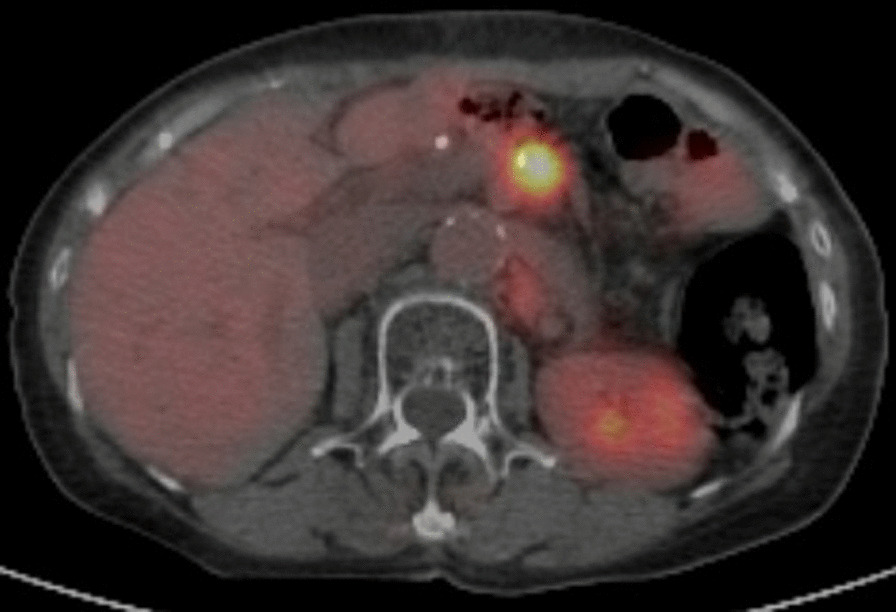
Positron emission tomography-computed tomography. Accumulation is observed in the nodule image
